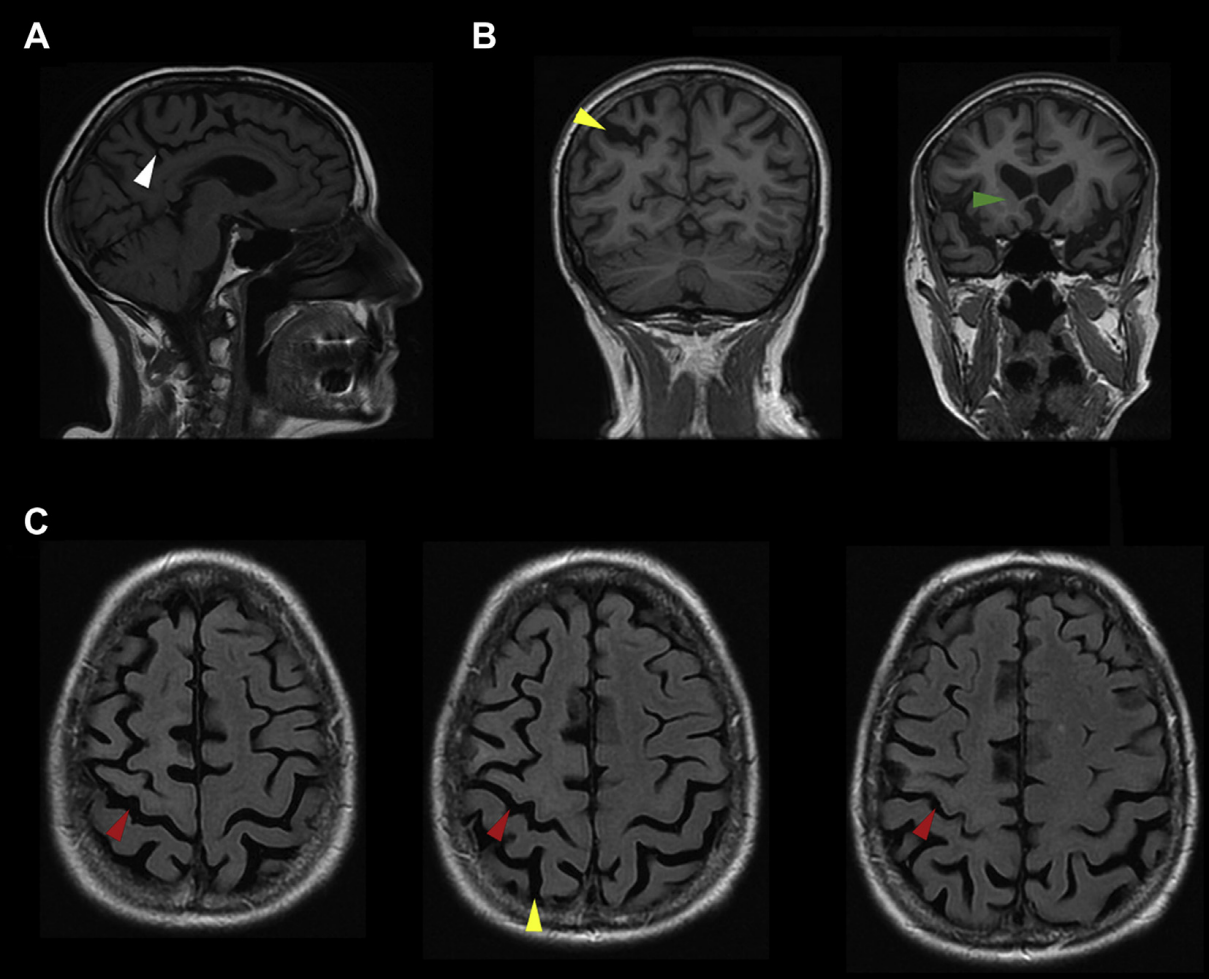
MRI brain scans of Patient SA. (A) Sagittal image demonstrating loss of volume of the left medial frontal and parietal cortex with a pathologically widened cingulate sulcus. (B) Coronal images showing loss of cortical volume particularly of the superior parietal lobe adjacent to a widened intraparietal sulcus, most prominently on the left, together with reduction in caudate head volume bilaterally. (C) Axial images demonstrating widened sulci over superior parietal and frontal regions, including the left central sulcus. White arrowhead = cingulate sulcus; yellow arrowhead = left intraparietal sulcus; red arrowhead = left central sulcus; green arrowhead = caudate head.
